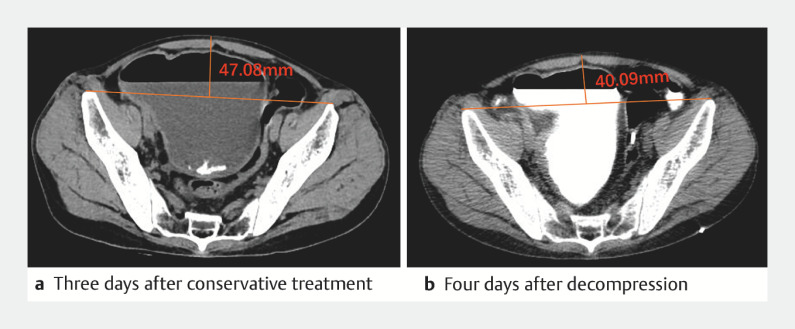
Computed tomography (CT) at the same level before and after decompression, after injecting 200 mL of contrast agent. The measurements show the vertical distance from the connecting line of the highest point of the bony landmarks on both sides to the highest point of the abdomen.
